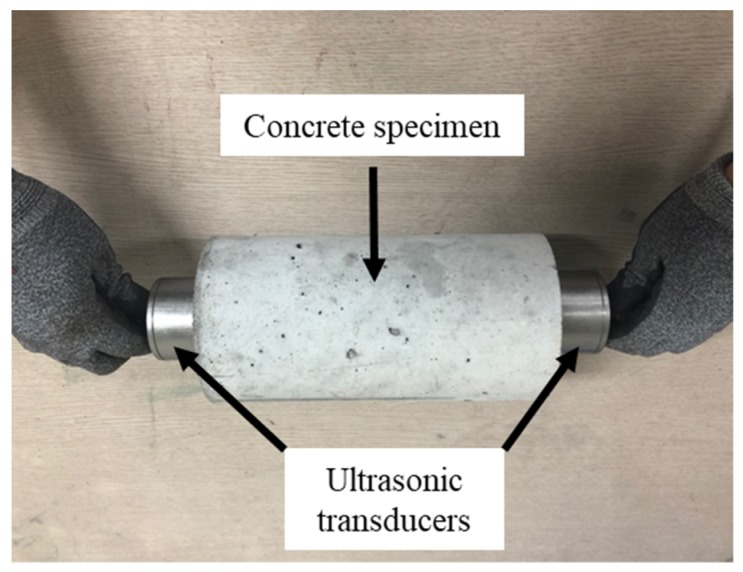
Measurement of ultrasonic velocity.
